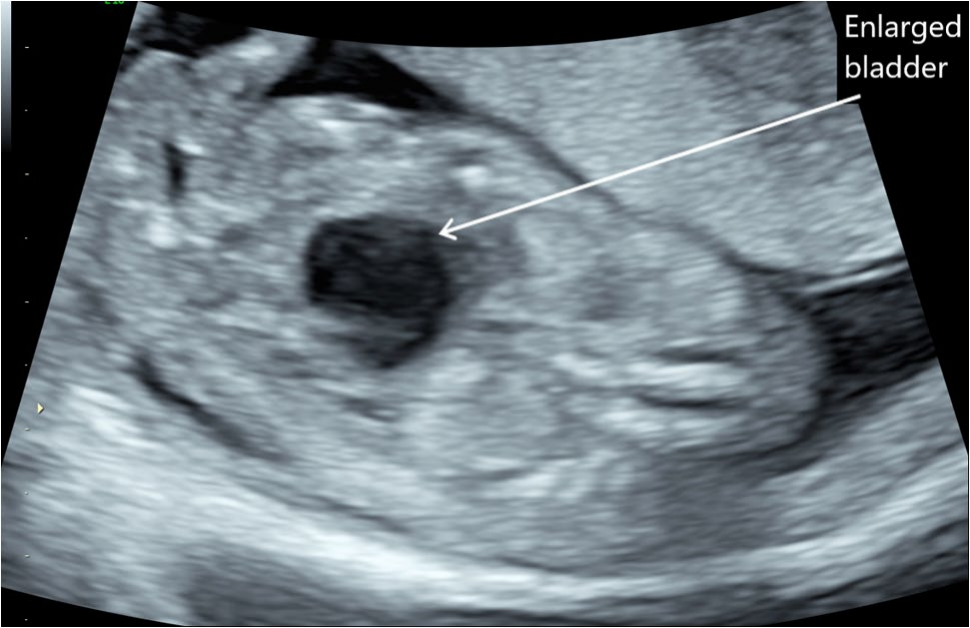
Antenatal ultrasound showing fetal megacystis (enlarged bladder) at 15/40 gestation
